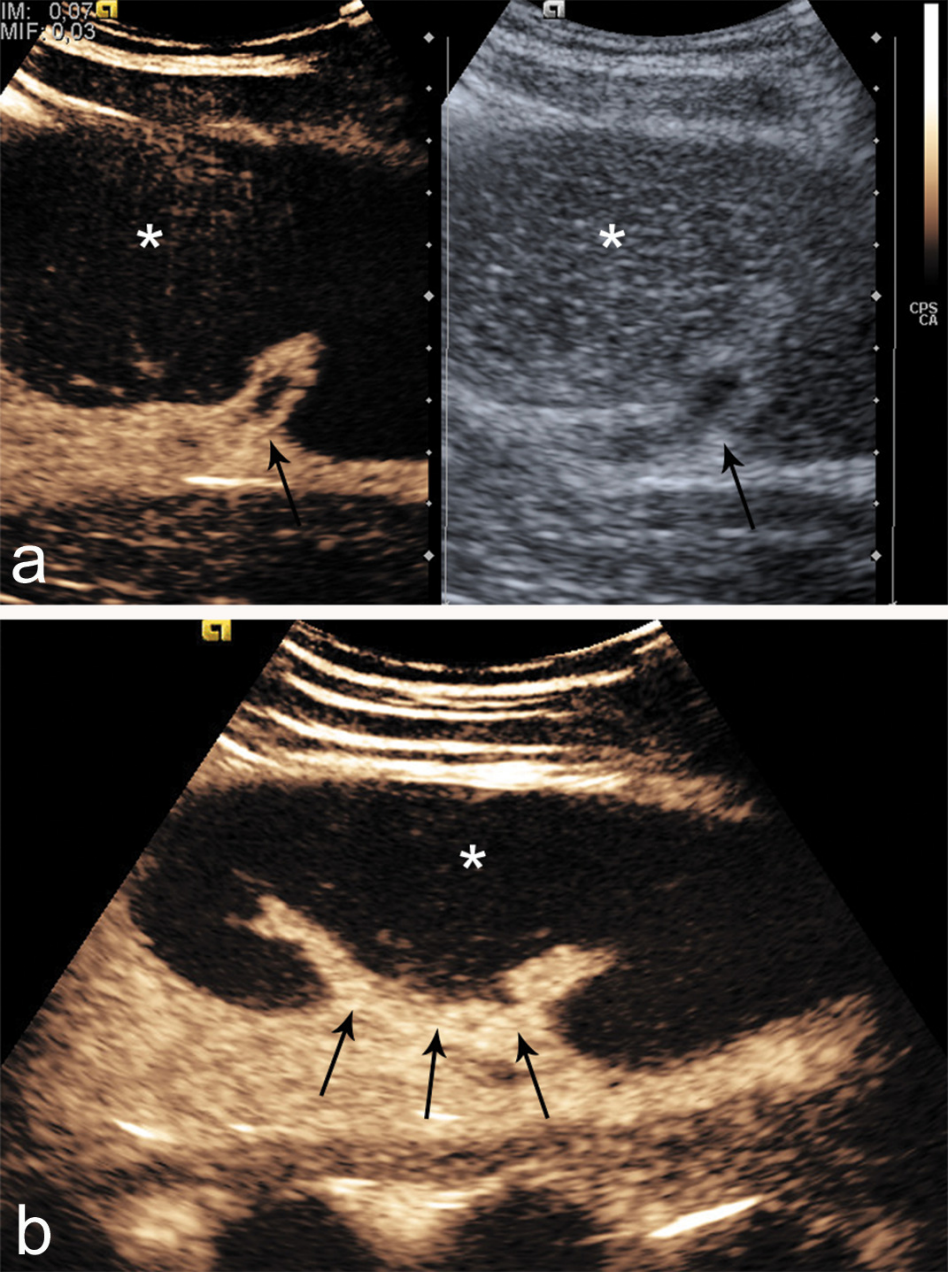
(a) 30 s sagittal contrast-enhanced ultrasound image showing lack of enhancement of the whole organ (asterisks), suggestive of infarction, there is absence of hilum enhancement (arrows). (b) It should be noted that no enhanced arterial or venous vessels are depicted in the splenic hilum (arrows).
